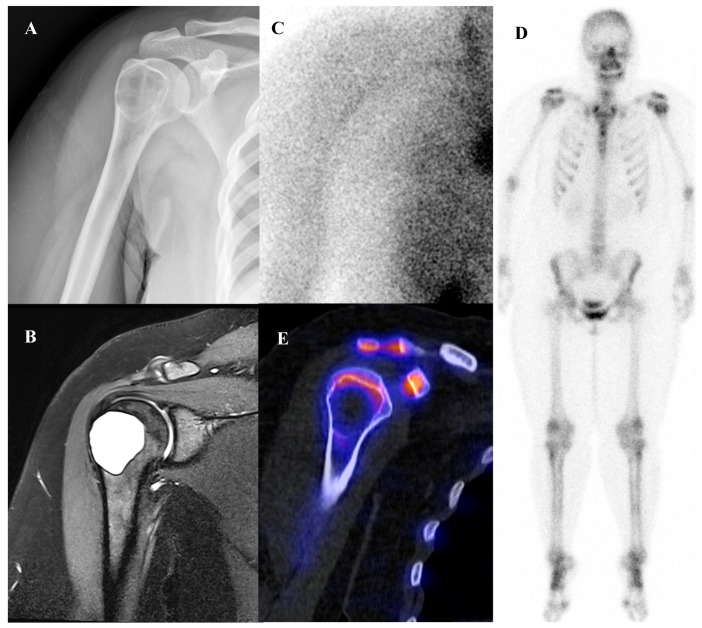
A 19-year-old female with a simple bone cyst of the right proximal humerus. (A) Plain radiograph demonstrates a well-defined lucent lesion. (B) T2-weighted fat-suppressed magnetic resonance imaging shows a lobulated, T2-bright cystic lesion at the metaphysis, adjacent to the physis, with thin rim enhancement and a T2 shine-through phenomenon. (C,D) Bone scintigraphy reveals no significant uptake in the right proximal humerus on either the blood pool or delayed phases. (E) Bone single-photon emission tomography/computed tomography similarly shows no significant tracer uptake at the lesion site. Growth plate shows mildly increased radiotracer uptake.
